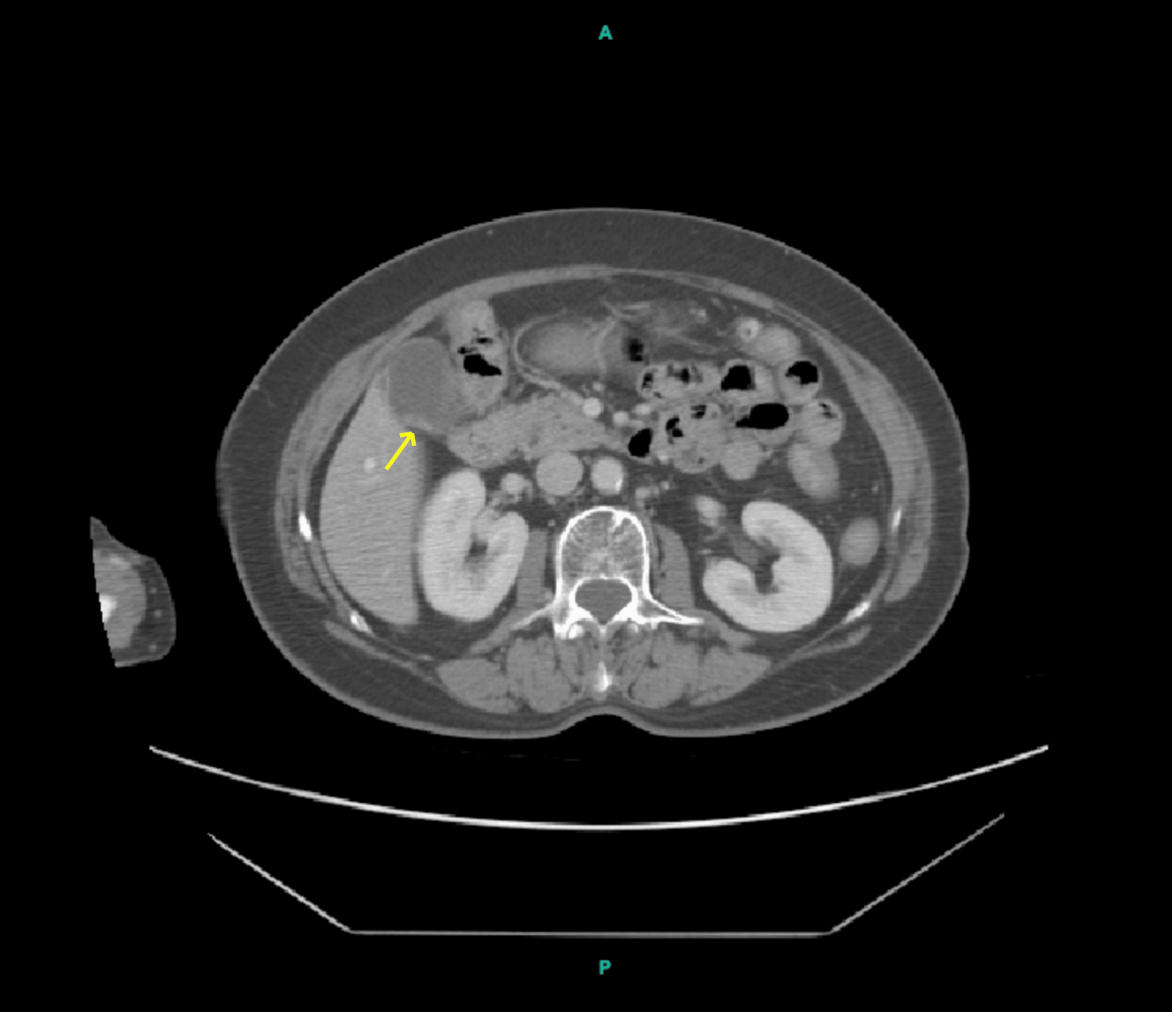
Axial CT scan of the abdomen demonstrating the gallbladder with multiple gallstones. The yellow arrow points to clustered hyperdense calcifications within the gallbladder lumen, consistent with gallstones.
